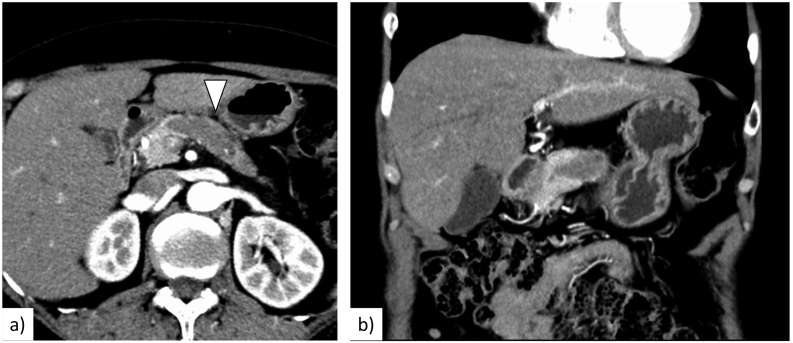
a. An enhanced computed tomography scan revealed a 46-mm low-density mass in the pancreatic body to tail (arrowhead). b. The tumour extended along the main pancreatic duct.
